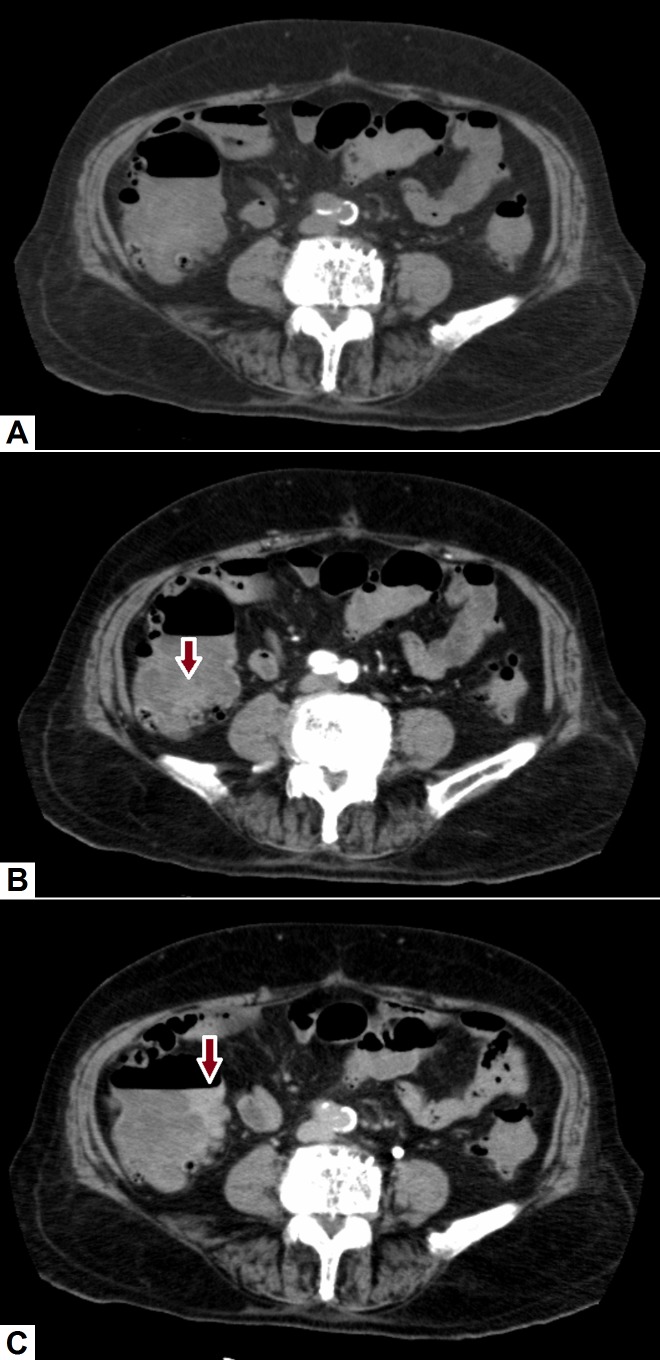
Active lower gastrointestinal hemorrhage. (A) Axial pre-contrast CT image shows slightly hyperdense material in the cecum (red arrow). (B) On arterial-phase axial image, there was an increase in the amount of hyperdense material within the cecum. (C) Axial delayed post-contrast image clearly shows active extravasation of contrast at the medial aspect of the caecum (red arrow). The patient then underwent catheter angiography for embolization of the culprit vessel (branch of ileocecal artery). CT: Computed tomography
